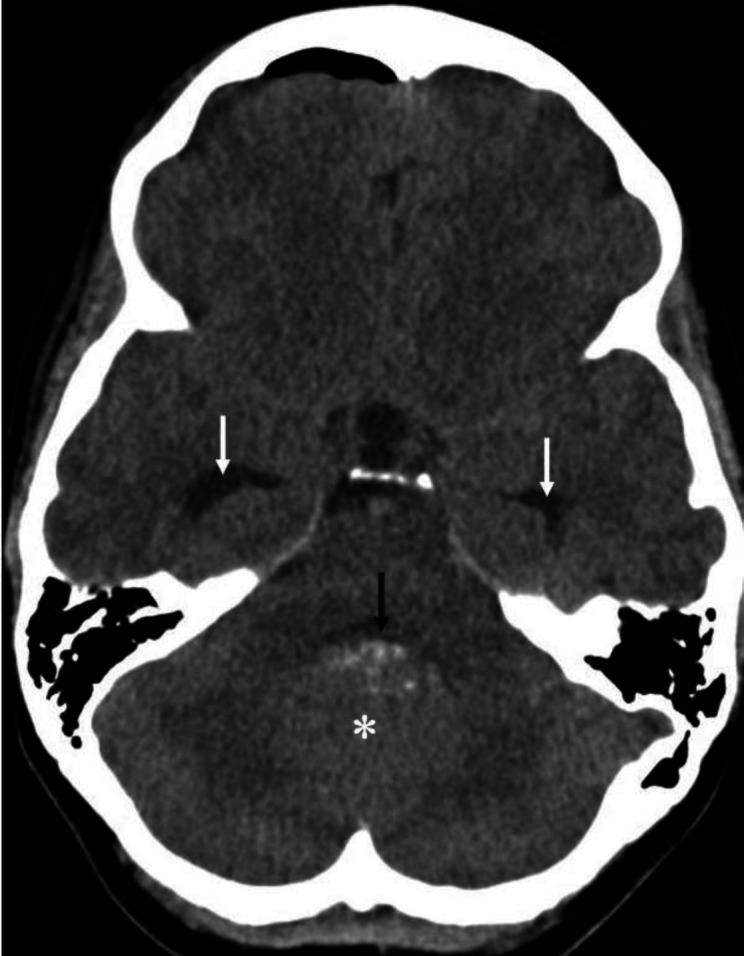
Non-contrast CT brain of a 11 year-old boy who presented with headache, vomiting and altered gait shows a slightly hyperdense midline posterior fossa mass (asterisk). High density areas seen in the anterior portion of the mass suggest calcification. Note the compression of the 4th ventricle (black arrow) by the mass. Cerebrospinal fluid hypodense areas (white arrows) in the bilateral temporal lobes are dilated temporal horns of the lateral ventricles and indicate increased intracranial pressure. The main differential diagnosis of a midline posterior fossa mass in a child is medulloblastoma
